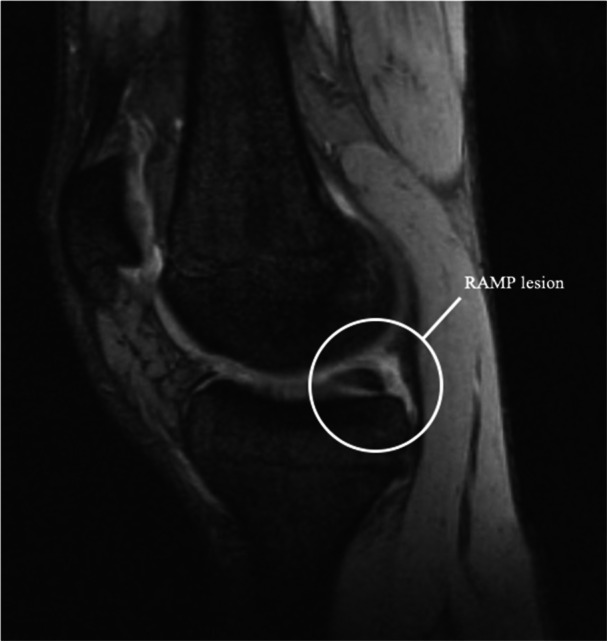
Magnetic resonance imaging (MRI) view: positive for RAMP lesion.
